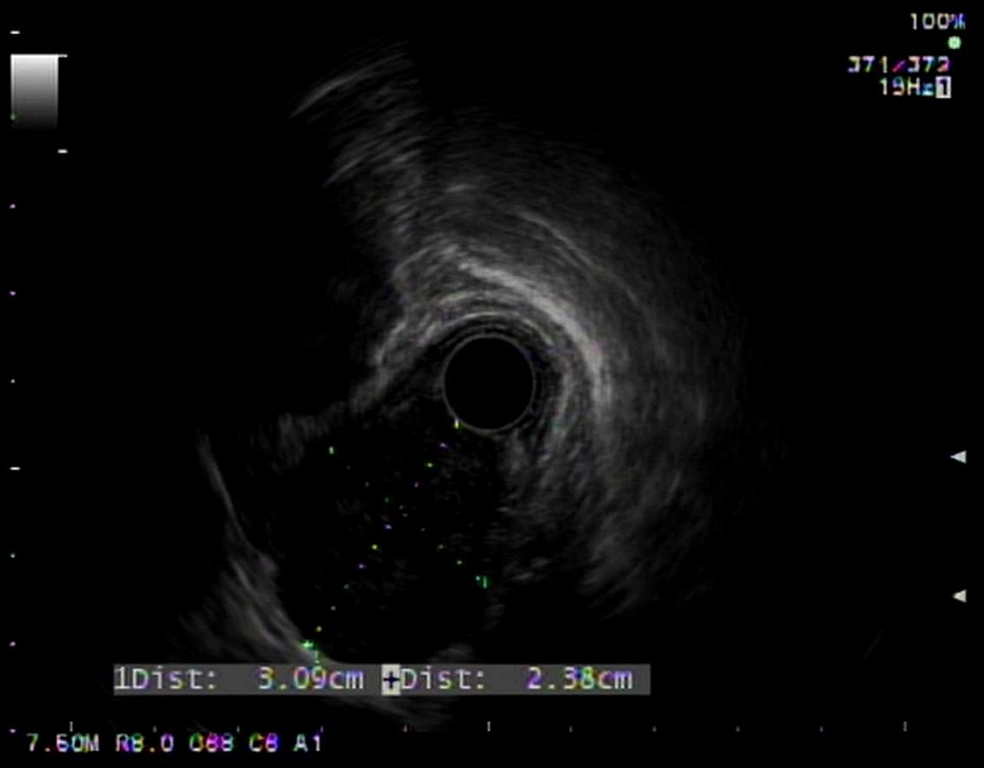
Initial endoscopic ultrasound view of the 3 cm hypoechoic lesion.
